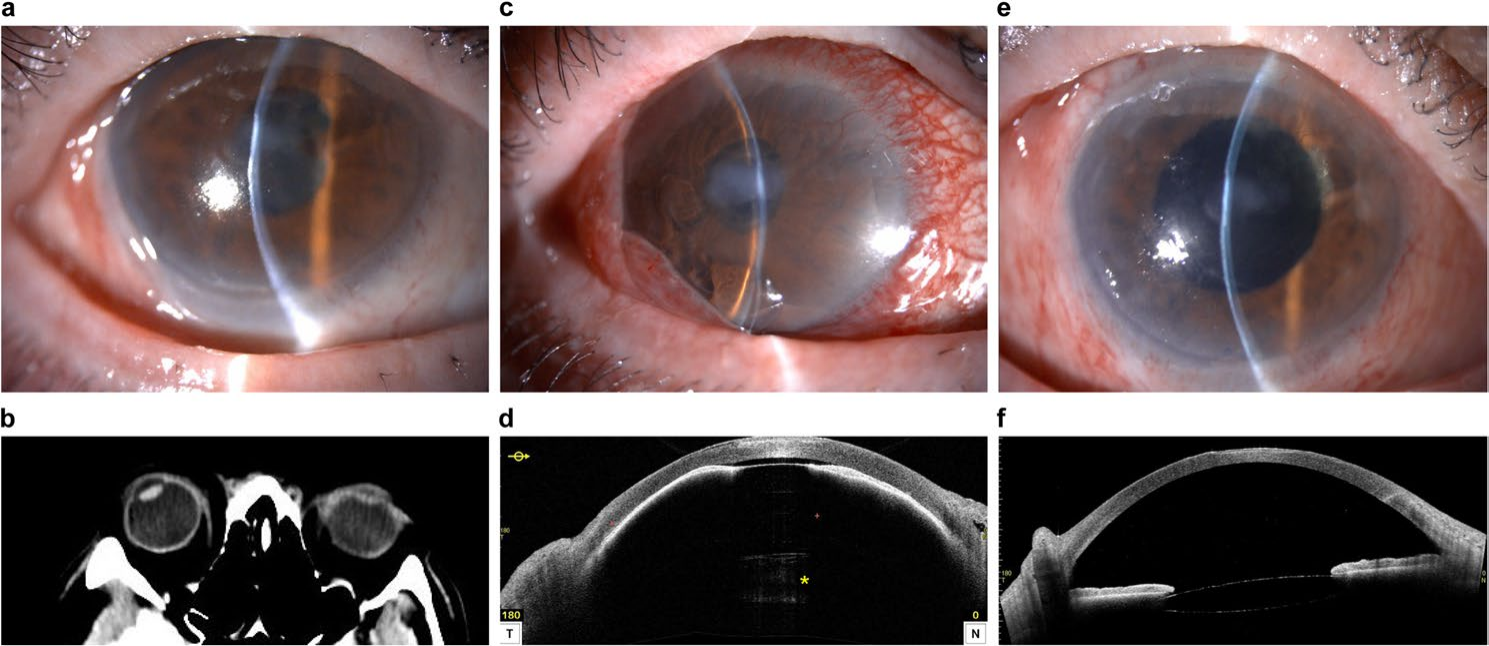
Pre- and Post-operative clinical findings of membrane pupillary-block glaucoma in a phakic eye. A. Slit-lamp examination at the initial visit to our hospital: a deep anterior chamber was observed. B. Computed tomography (CT) image taken in the emergency room one month after the initial visit showed that the cataractous lens was in its original position. C. Slit-lamp photograph taken on the same day as (B) showed an extremely shallow anterior chamber and marked conjunctival hyperemia. The pupillary zone details were obscured due to corneal opacity. D. Anterior segment optical coherence tomography (AS-OCT) image taken on the same day as (B) revealed an extremely shallow anterior chamber and the presence of a pupillary membrane. No lens displacement was observed (*). E. Slit-lamp photograph one month postoperatively showed a deep anterior chamber. F. AS-OCT image taken on the same day as (E) also showed a deep anterior chamber postoperatively
